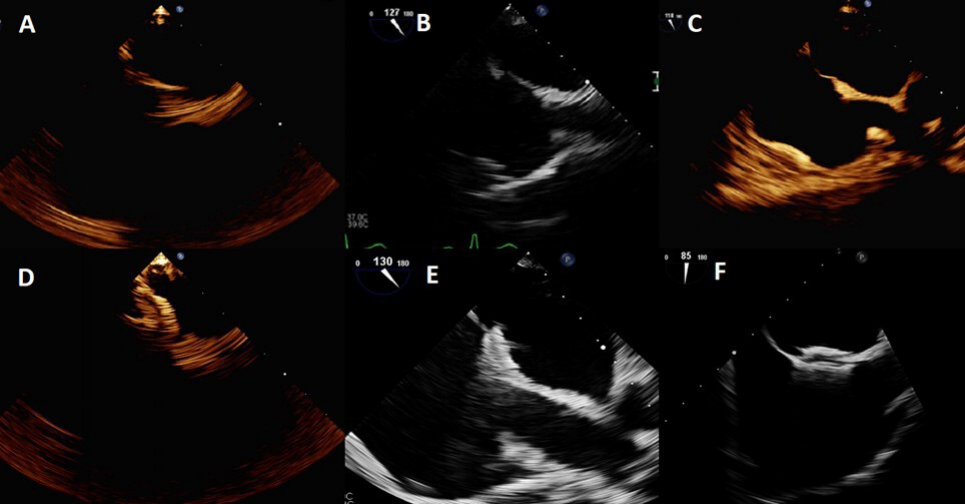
MicroTEE-probe image quality. The image quality of transoesophageal echocardiography with the microTEE-probe varied from poor (A, D) to acceptable (B, E) and good (C, F). (A–C) Bicaval images taken at the beginning of the procedure. (D–F) Acquired at the end of the procedure with the PFO occlude device in its final position. A poor image quality was characterised by blurry structures and large pixels while some structures were not visible at all (A, D). The image quality was good enough for device implantation, but detailed analyses was not possible. With acceptable image quality (B, E) most structures were visible, and structures in the near field could be studied with some details. A good image quality (C, F) was characterised by clear structure borders and resolution of the details near ordinary transoesophageal probe. PFO, patent foramen ovale; TEE, transoesophageal echocardiography.
